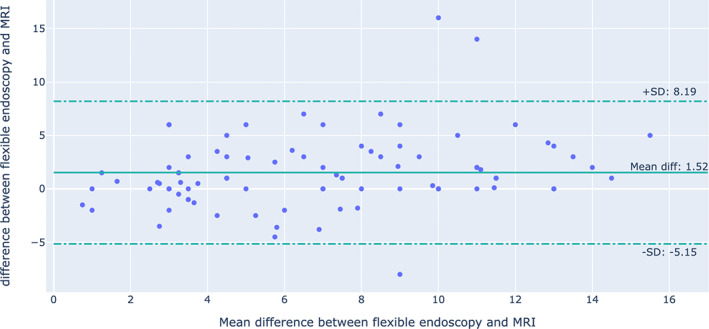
Bland Altman Plot to visualize the differences in measurements between MRI and endoscopy. The sold line represents the average difference in measurements. The dotted lines represent the upper and lower limits of the 95% confidence interval for the average difference
